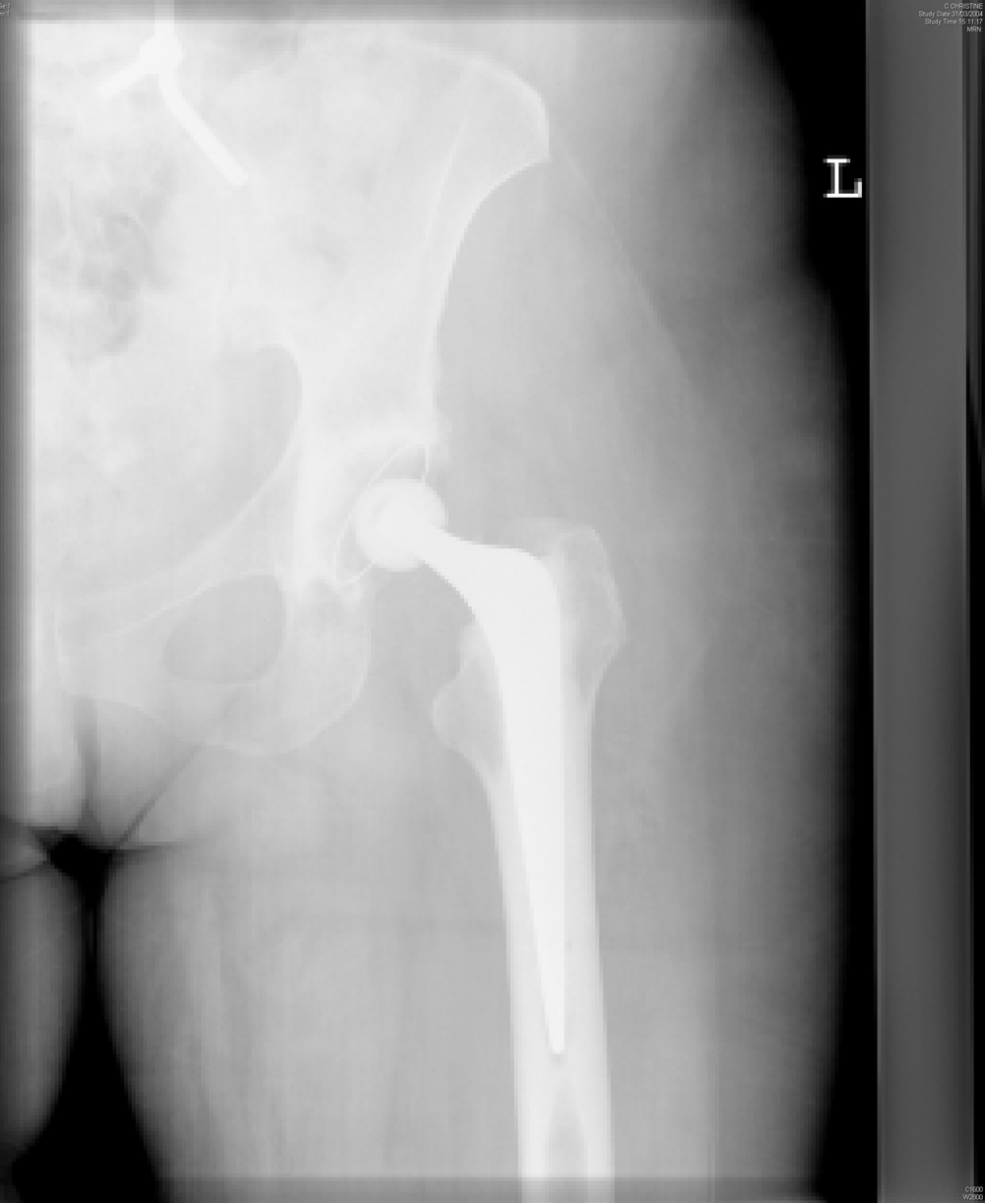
Post-reduction anterior-posterior radiograph of the hip arthroplasty.
